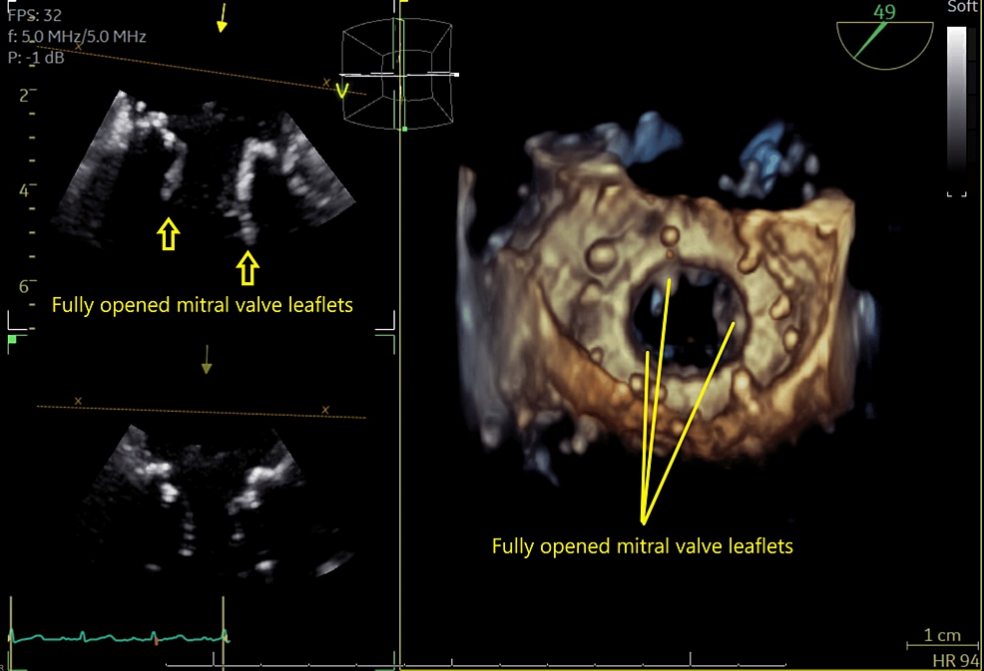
Preoperative echocardiographic depiction of the mitral valve. The Sapien 3 Ultra valve in the mitral position demonstrates preserved leaflet excursion. The yellow arrows and lines show fully opened mitral valve leaflets.
